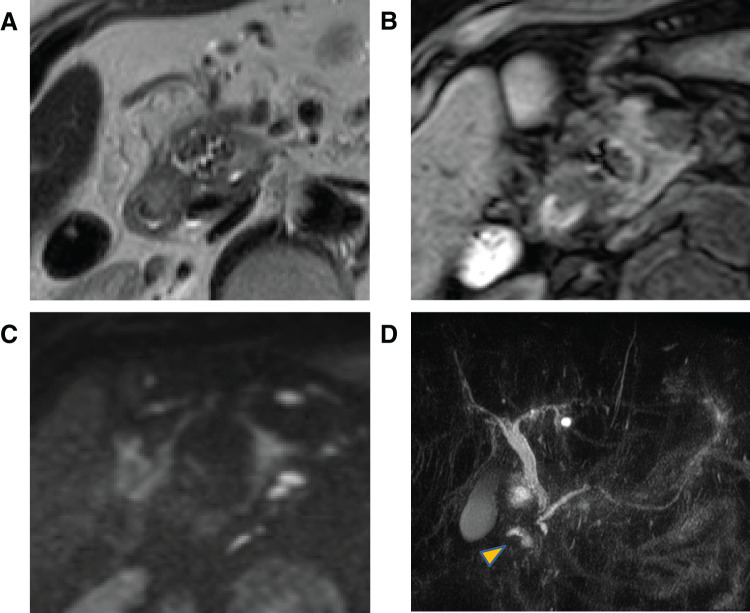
MRI images. (A) Image obtained using T2-weighted MRI. (B) Image obtained using T1-weighted MRI. (C) Image obtained using DWI. (D) MRCP image (arrowhead: mass). The groove area mass exhibits internal characteristics of high signal, low signal, and high signal intensity during T2-weighted, T1-weighted, and DWI imaging, respectively. Additionally, small clustered nodules exhibiting low signal intensity during T2-weighted MRI, T1-weighted MRI, and DWI are observed. MRCP reveals no dilation or irregularity in the main pancreatic duct. Pancreaticobiliary maljunction is not observed. Gallbladder and common bile duct stones are absent. Tapering of intrapancreatic bile duct is observed. DWI, diffusion-weighed imaging; MRCP, magnetic resonance cholangiopancreatography
